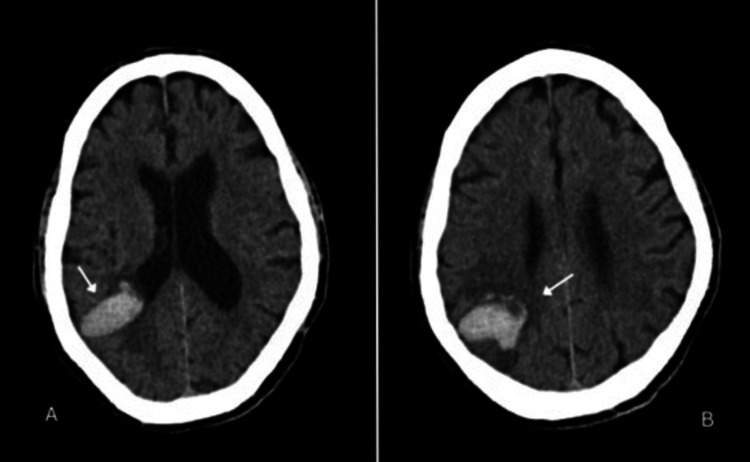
Computed tomography without contrast Axial non-contrast computed tomography (CT) scans of the head (A, B) demonstrating a 5.2 cm right parietal intraparenchymal hemorrhage with surrounding edema (arrow) and a 3 mm leftward midline shift. There is an additional right temporal intraparenchymal hemorrhage measuring 1.0 cm.
